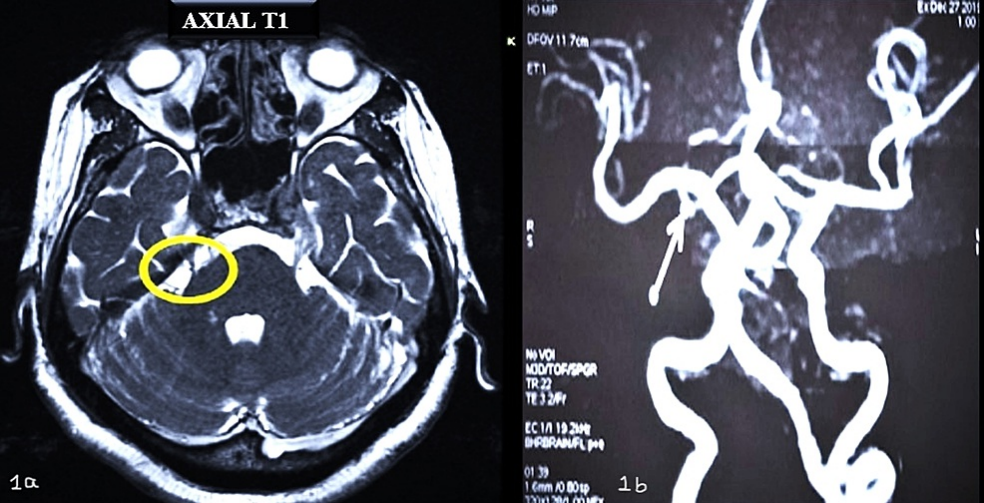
MRI (1a) and MRA (1b) findings show a vascular loop from the right superior cerebellar artery that was abutting and indenting the right trigeminal nerve at the cisternal segment and root entry zone MRI: magnetic resonance imaging; MRA: magnetic resonance angiography
